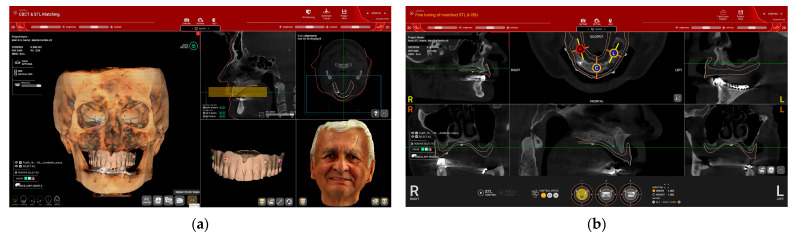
(Patient#1): (a) DICOM files from the CBCT, STL files from the intraoral scan (edentulous maxilla with preexisting adapted denture), and OBJ files from facial scanning were imported into the software; (b) fine manual alignment of the DICOM and STL files.
